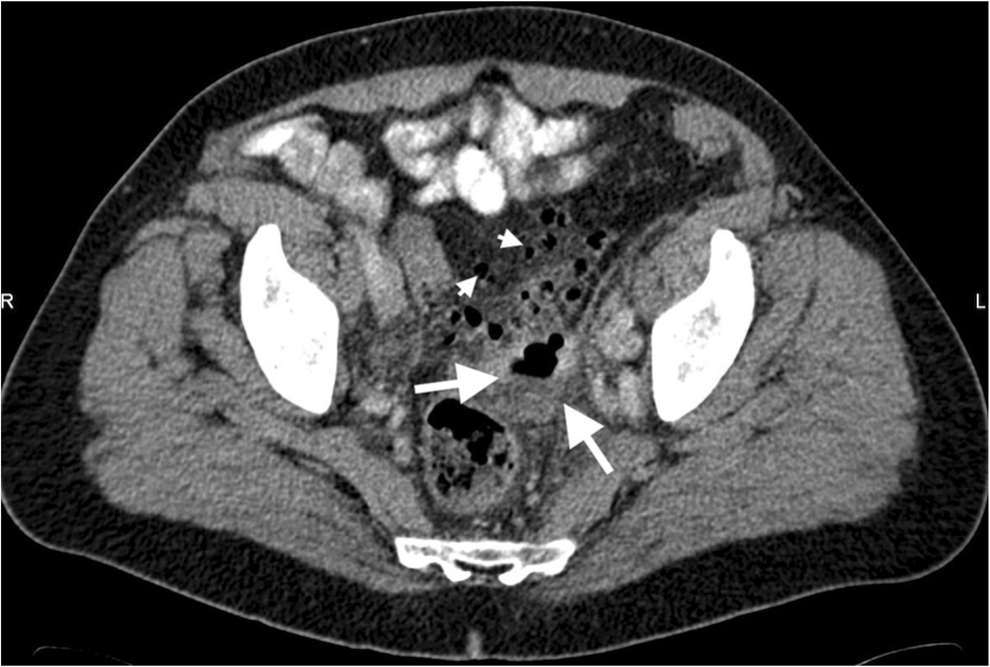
Thirty-eight year-old male with diverticulitis and well-contained perforation. Axial contrast-enhanced CT demonstrates edema and thickening of the sigmoid colon wall with multiple diverticulums. A fluid collection adjacent to the sigmoid colon (*arrows*) is an abscess caused by perforation of the diverticulitis. Free air pockets (*arrowhead*) confined to the pericolonic region are seen
